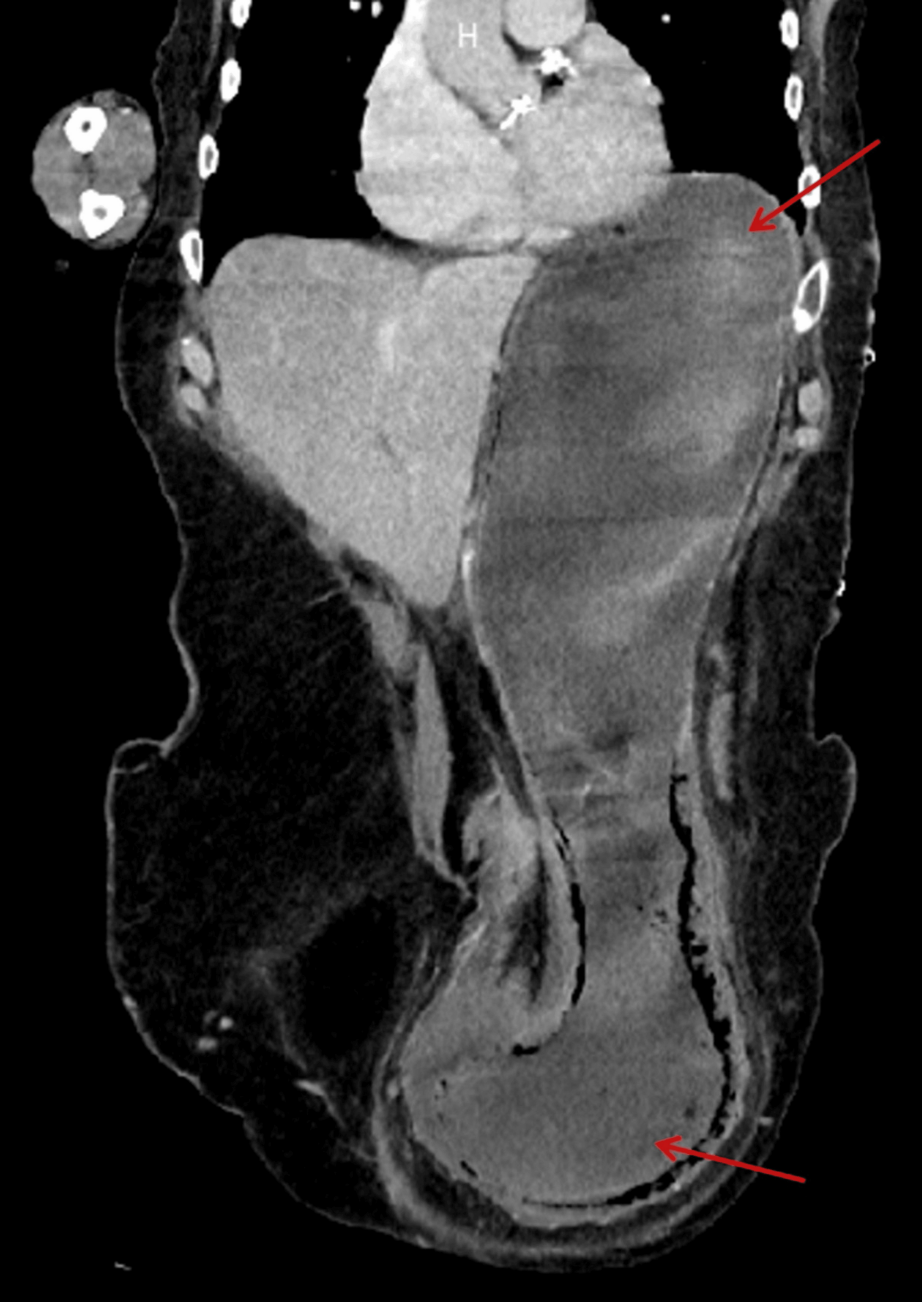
CT imaging of the abdomen and pelvis in a coronal view. Red arrows indicate the descent of the stomach into the inguinoscrotal hernia sac. CT: computed tomography
